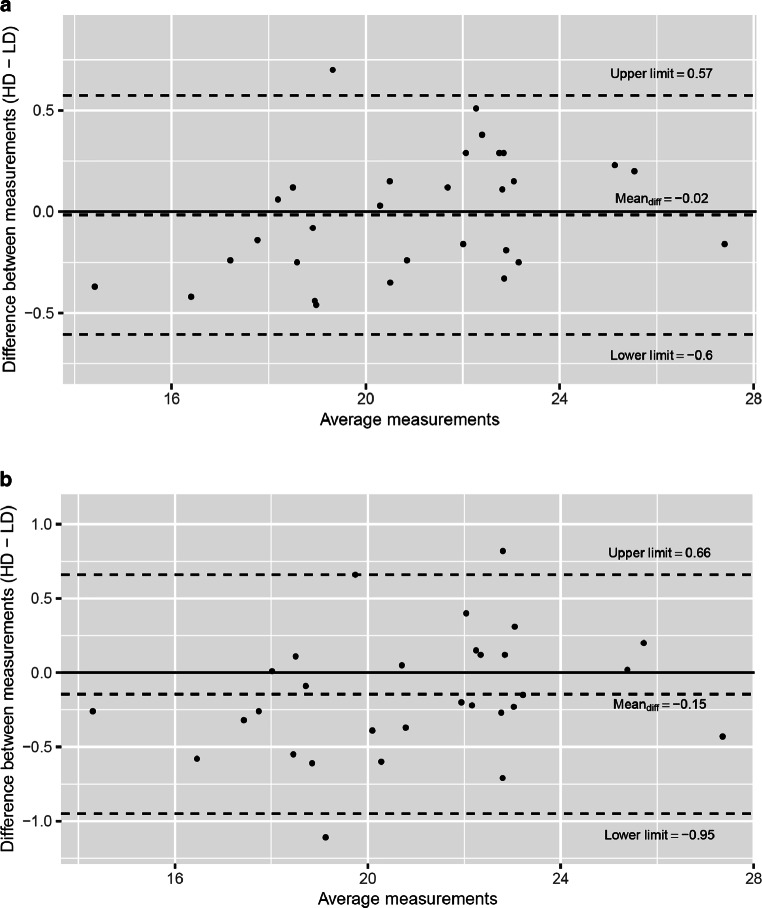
Bland–Altman plots of low-dose (LD-CBDT) and high-dose cone-beam computed tomography (HD-CBCT) tooth length measurements (mm) from a the first round of measurements and b the second round of measurements Bland-Altman-Plots der Zahnlängenmessungen (mm) mit der dosisreduzierten (LD-) und der high-dose digitalen Volumentomographie (HD-CBCT) a aus der ersten und b aus der zweiten Messrunde
